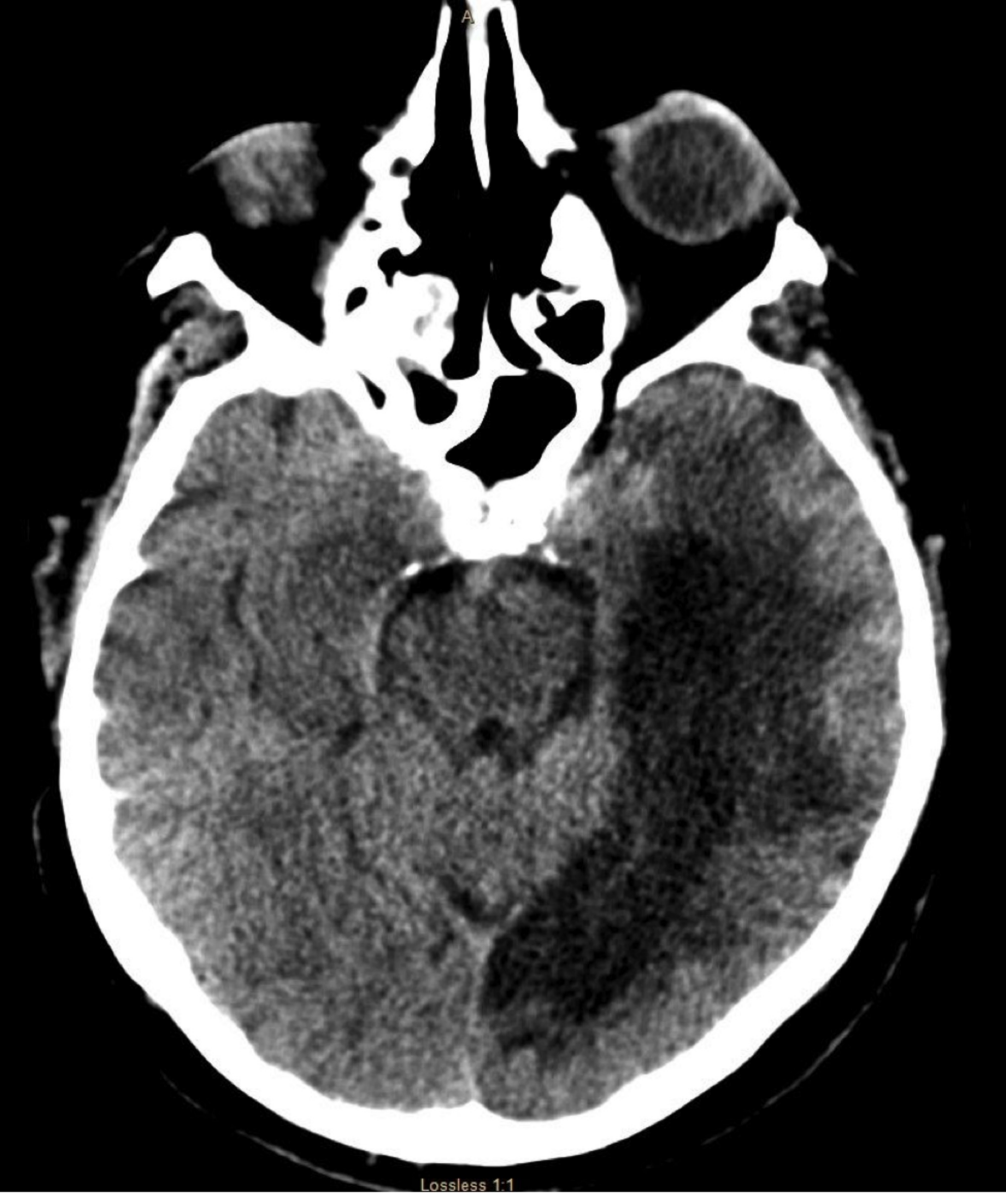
CT scan of the head that shows no acute changes. There is an area of hypodensity in the right temporal region, consistent with patient know prior history of left PCA embolic stroke
